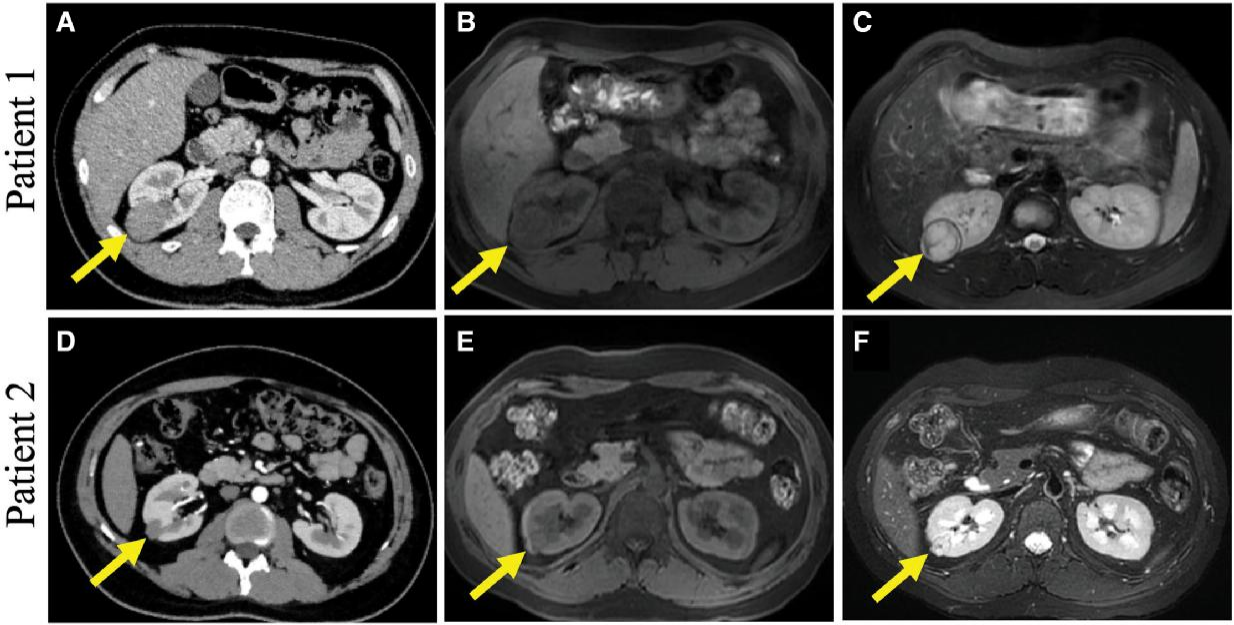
CT and magnetic resonance imaging of two patients. (*A*) Enhanced CT scan showed a round-shaped mass, with no apparent enhancement in the upper pole of the right kidney. (*B–C*) Renal magnetic resonance imaging showed an iso-signal mass in the T1-weighted image and a heterogeneous mass on the T2-weighted image. (*D*) The CT scan showed an irregular nodule in the mid- to back outer side of the right kidney, with no enhancement on the contrast-enhanced scan (arrow). (*E–F*) The mass showed iso-signal intensity and a heterogeneous signal intensity in the T1- and T2-weighted magnetic resonance imaging.
